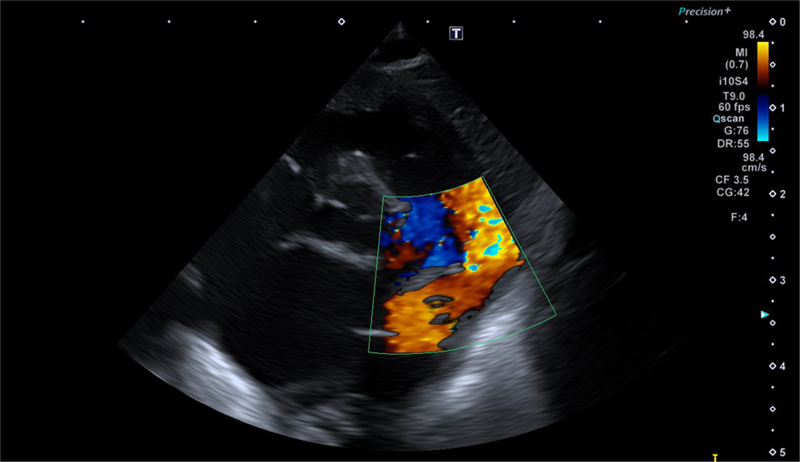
Echocardiogram (ductal view, color Doppler) showing partial obstruction of the ductus arteriosus due to the vegetation.
